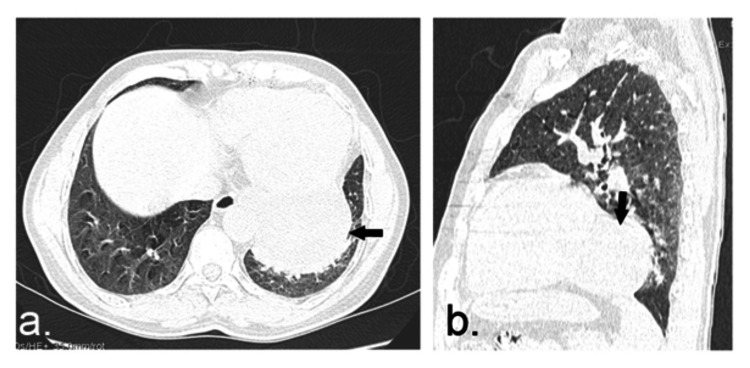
(a) axial and (b) sagittal unenhanced CT (lung window) images show abnormal, round-oval lesion (black arrows) along the left posterior cardiophrenic angle
